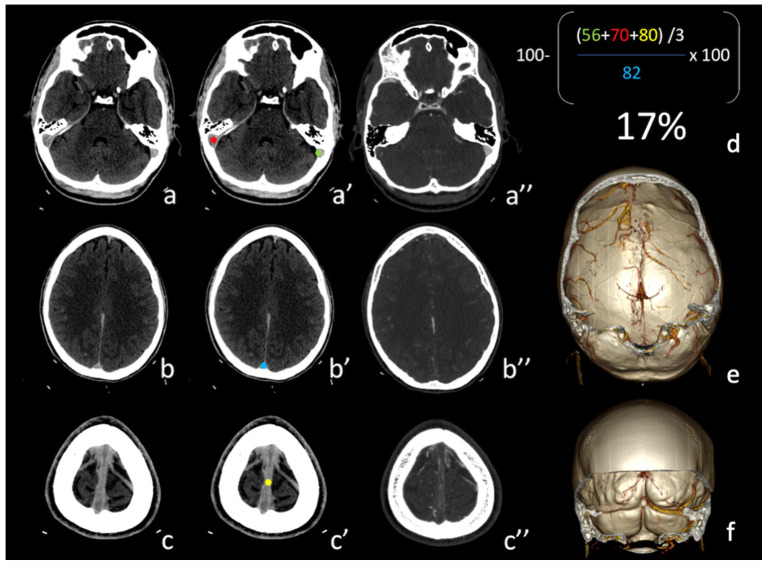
(a–c) Non-contrast CT (NCCT) images of a patient with superior sagittal sinus and right transverse sinus thrombosis; (a’–c’) example of the proposed method applied to the patient’s cerebral venous system with the proposed formula (d) indicative of venous thrombosis; (a”–c”,e,f) CT angiography that confirms superior sagittal sinus and right transverse sinus thrombosis.
